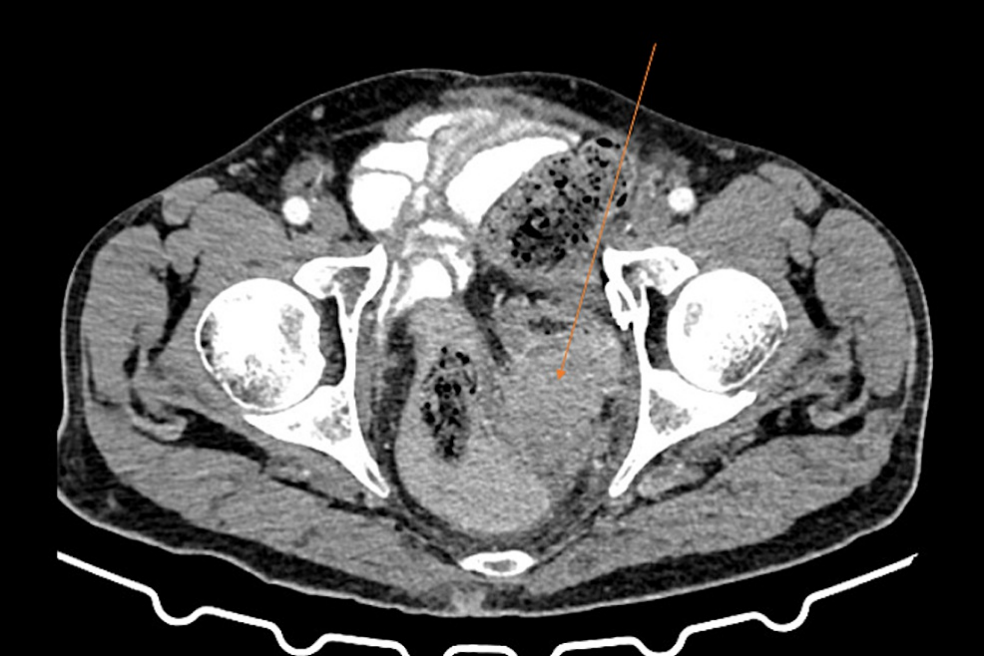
Axial section of contrast CT of the abdomen. The arrow shows a mass lesion in the sigmoid colon and involves the left obturator space.
